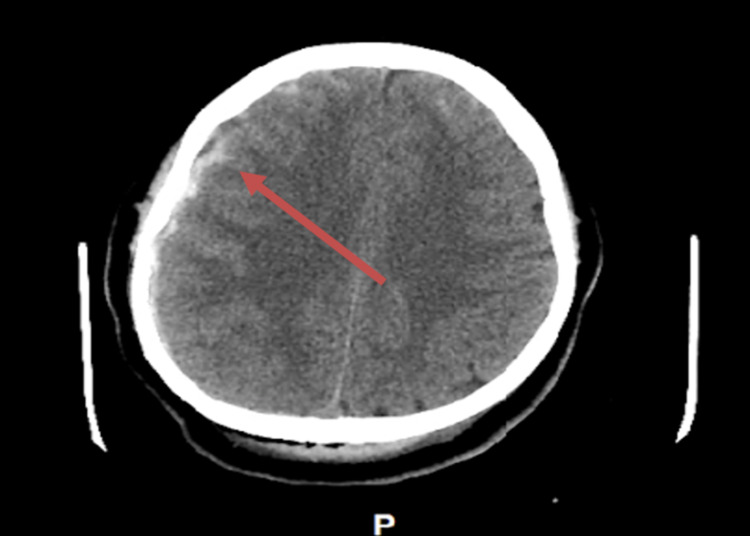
Initial brain CT showing subdural hematoma (arrow)
